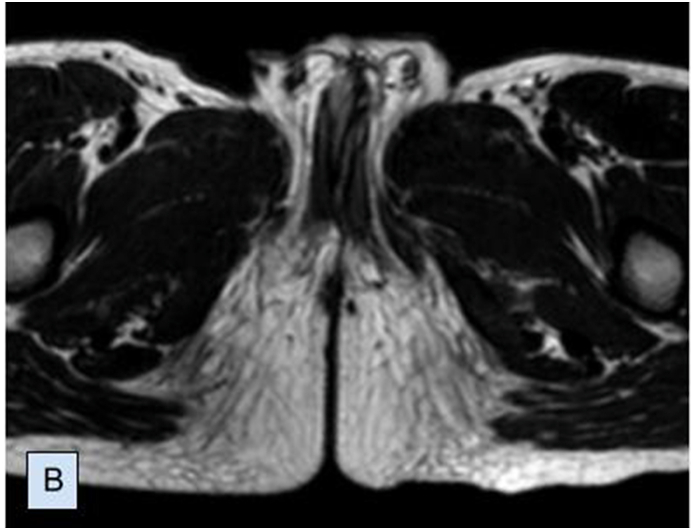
MRI study of the pelvic organs including penile structures.
